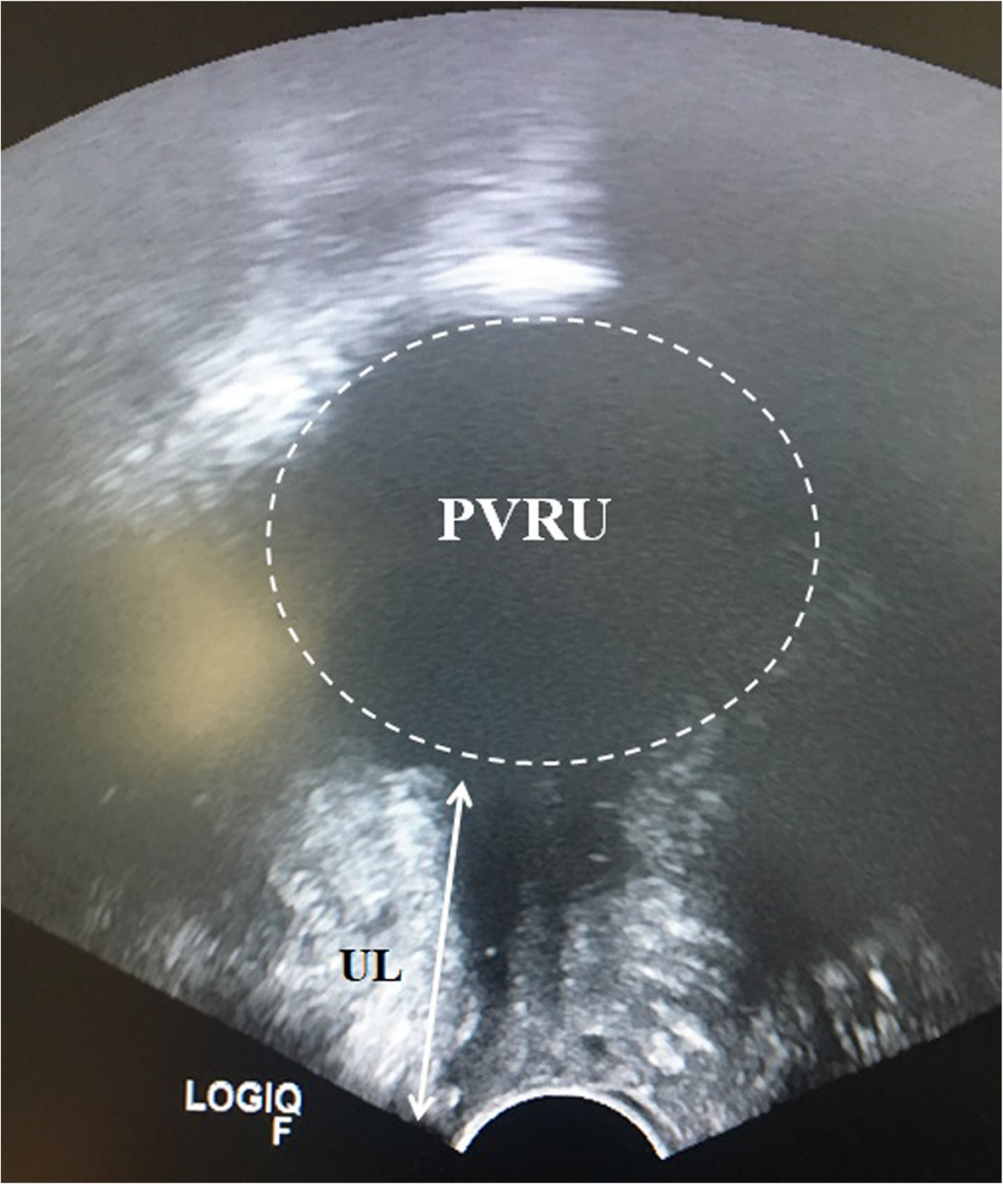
Vaginal ultrasound scan image of the urinary bladder after an attempt to completely empty the bladder. The examination was carried out with a LOGIQ S7^®^ ultrasound machine (GE Healthcare, Chicago, IL, USA). PVRU represents the post-void residual urine volume (hyperechoic), inside the urinary bladder (*dashed white line*), while UR is the urethral length (*arrow* - 3.66 cm). *PVRU* post-void residual urine
